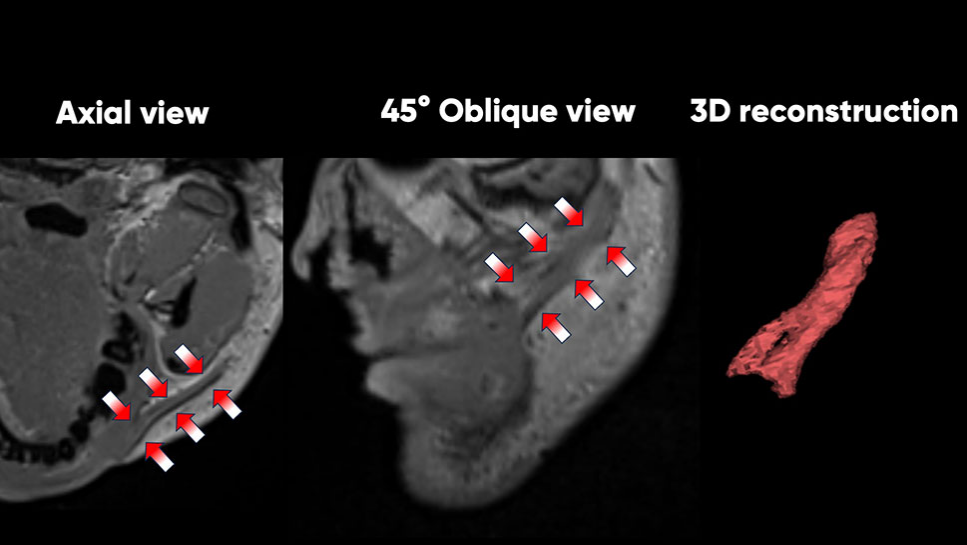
Representation of the multiplanar reconstruction from which the slice was highlighted and measured for the zygomaticus major muscle. The location and method of MRI slice acquisition are shown by the red rectangle superimposed on a facial model
